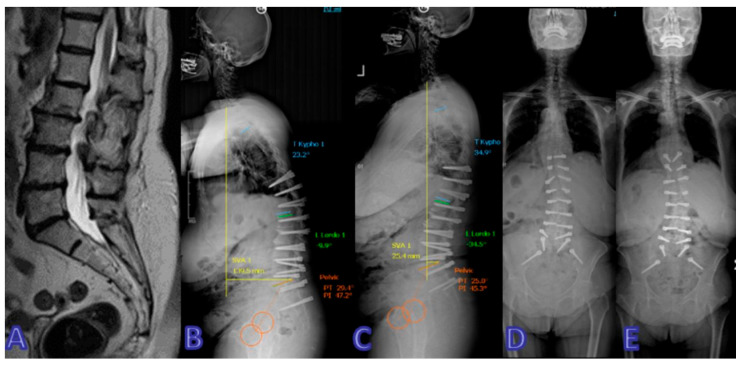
A 69-year-old woman underwent surgery for L4–5 stenosis 10 years ago and presented with progressive forward bending and low-back pain. (A) Preoperative MRI image. (B) Lateral X-ray image after the first stage (prior to rod placement). (C) Lateral X-ray image after the first stage (following rod placement). (D) Anteroposterior X-ray image after the first stage (prior to rod placement). (E) Anteroposterior X-ray image after the first stage (following rod placement). Significant improvement was noted in both clinical findings and spinopelvic parameters postoperatively.
